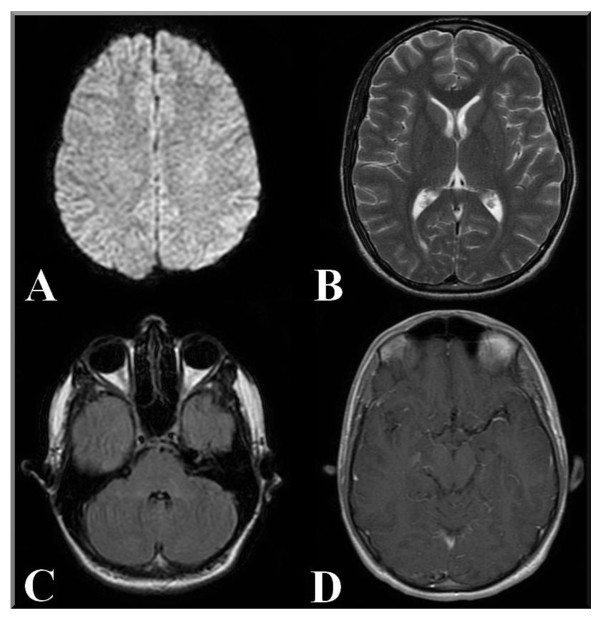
MR imaging of the patient at follow-up 3 weeks later. DWI remains the same, without any signal alteration (A). On the Axial T2 FRFSE image, we can see substantial regression of the previous lesions and no new lesions (B). The Axial T2 FLAIR image shows that the fourth ventricle has returned to its normal size. All signal intensities are normalized (C). On the T1W Axial FLAIR there is no enhancement of cerebral vessels after contrast medium was administered, as described above (D).
